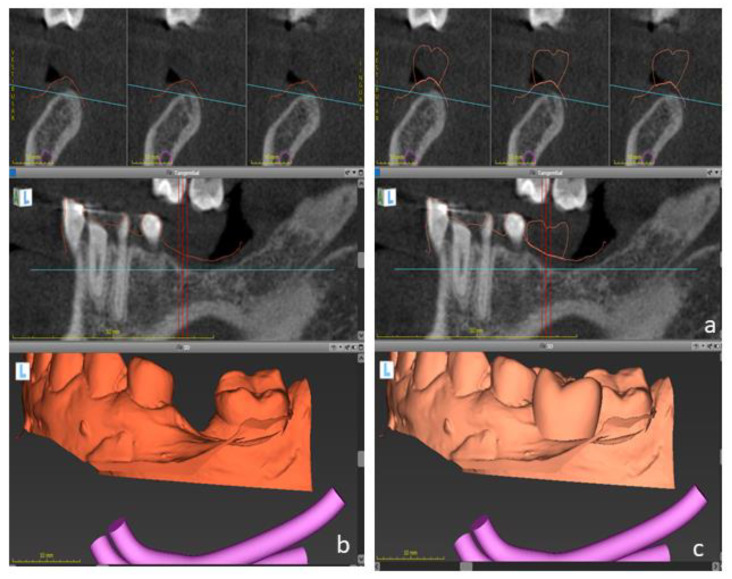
Dental implant planning on lower jaw. Sagittal cuts of cone-beam computed tomography (DICOM files) are shown (a). DICOM files were superimposed to the intraoral casts (b) and to the digital wax-up (c) to determine the optimal implant position.
